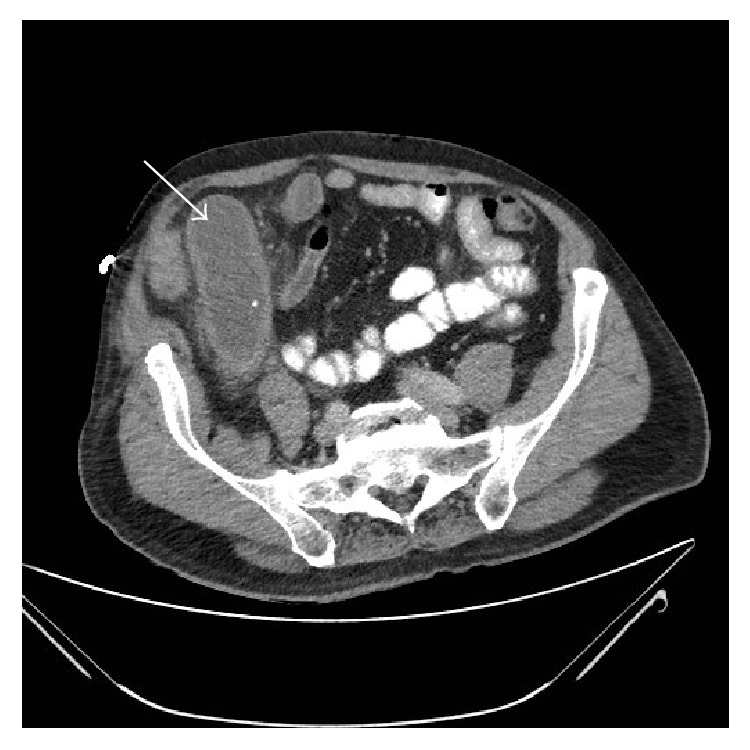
CT abdomen pelvis on admission showed a change in the morphology of the tubular cystic structure which was now vertical to transvers axis orientated with thickened walls and fat stranding suggestive of torsion or inflammation of the mucocele.
